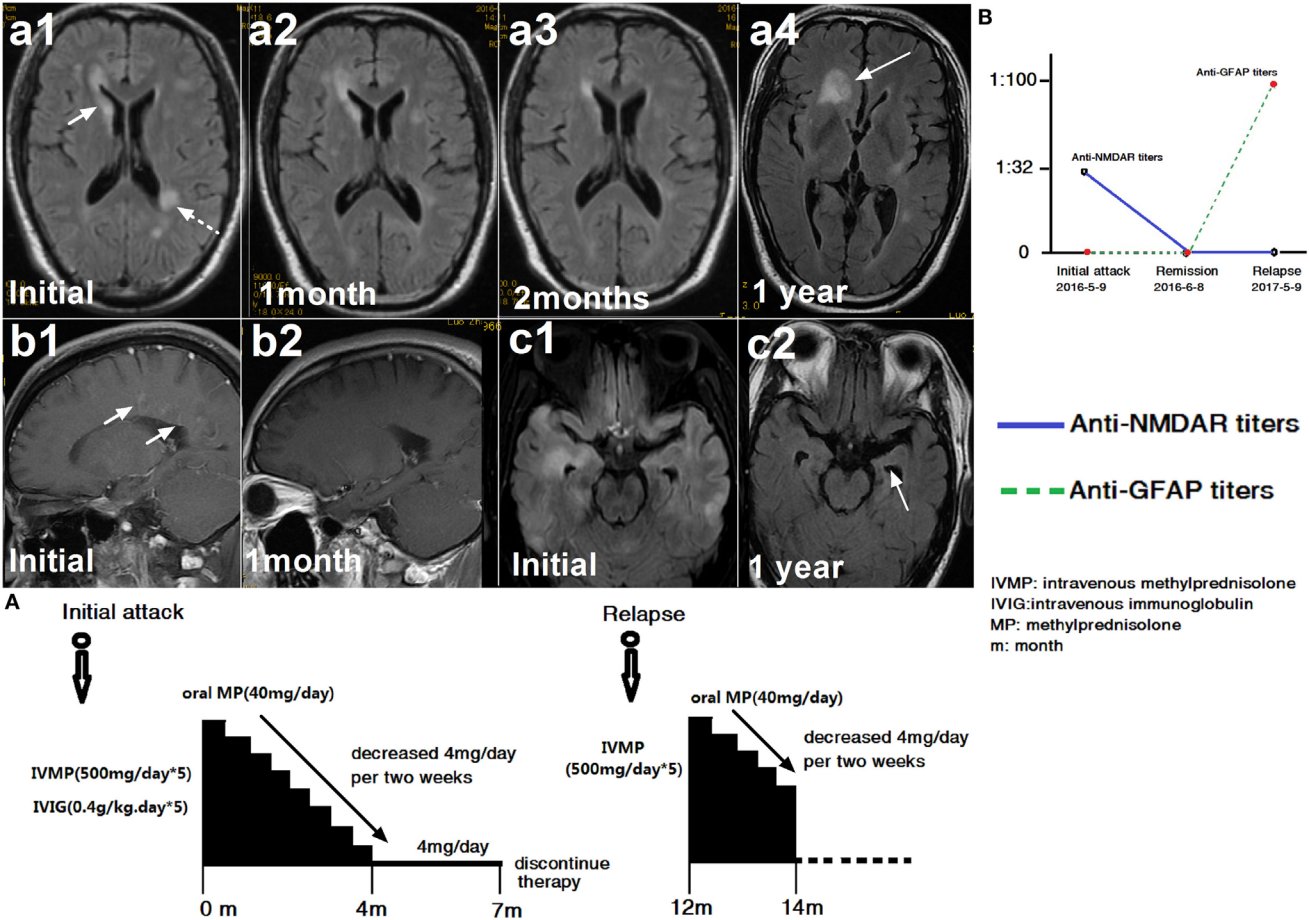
A patient with glial fibrillary acidic protein (GFAP) astrocytopathy following anti-*N*-methyl-d-aspartate receptor (NMDAR) encephalitis. (a1–c2) Brain magnetic resonance imagings (MRIs). (A) Treatment course. (B) NMDAR and GFAP antibody titers. The first brain MRI showed lesions in the right caudate nucleus, white matter along the ventricle (a1) and enhancement around vessels (b1). At this time, cerebral spinal fluid (CSF) showed positive NMDAR-IgG at 1:32 titer. The patient was treated with intravenous methylprednisolone (500 mg/day for 5 days) and intravenous immunoglobulin (0.4 g/kg for 5 days). At follow-up, an MRI scanning showed improvement in the brain abnormalities (a2, a3) and the disappearance of enhancement (b2). One month later, NMDAR-IgG and GFAP-IgG were negative. However, the patient relapsed 1 year later due to discontinuation of oral steroid. At this time, the MRI showed a large lesion in the frontal lobe (a4). Compared with the initial MRI scan, left hippocampal atrophy (c1, c2) was found. At this time, CSF was positive for GFAP antibody but negative for NMDAR antibody (B).
